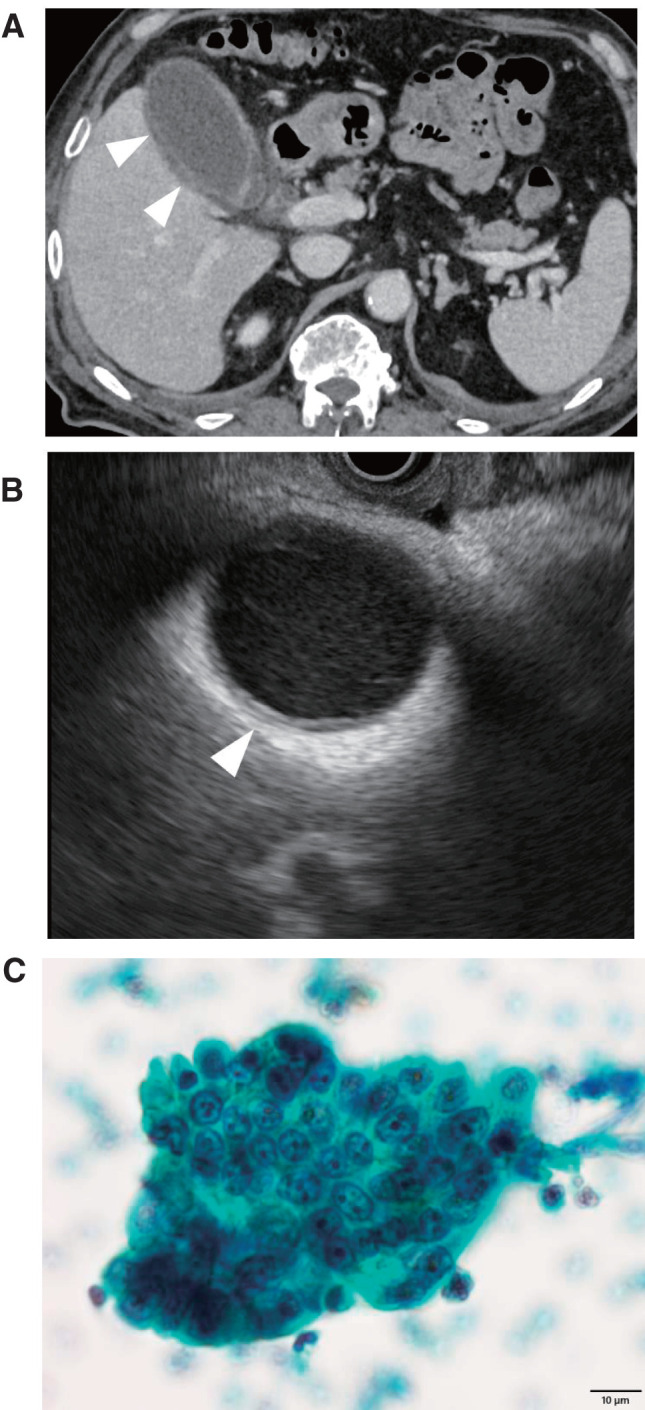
Imaging and cytology at initial admission. (A) Axial CT showing gallbladder wall thickening (white arrowheads). No bile duct dilation or tumor was observed. (B) EUS demonstrating gallbladder wall thickening (white arrowheads), consistent with CT findings. No tumor was detected. (C) Cytology of bile juice showing adenocarcinoma. Scale bar: 10 μm. EUS, endoscopic ultrasonography
